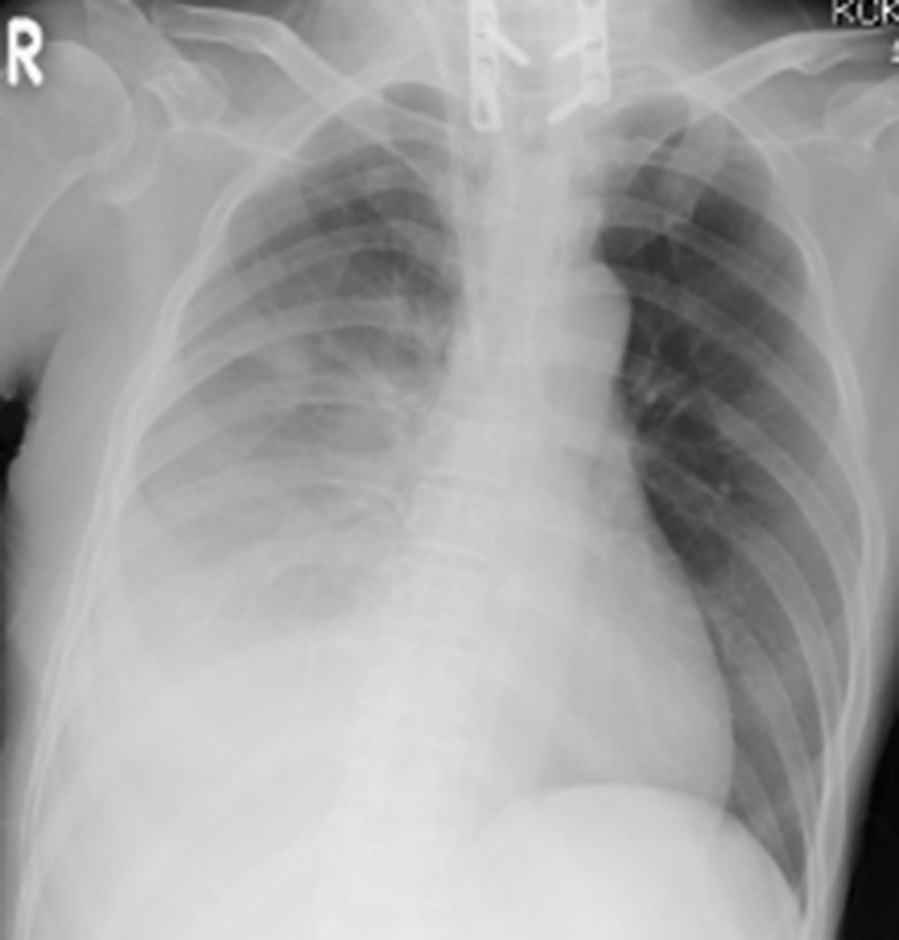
Chest radiograph taken before surgery shows right hydrothorax
